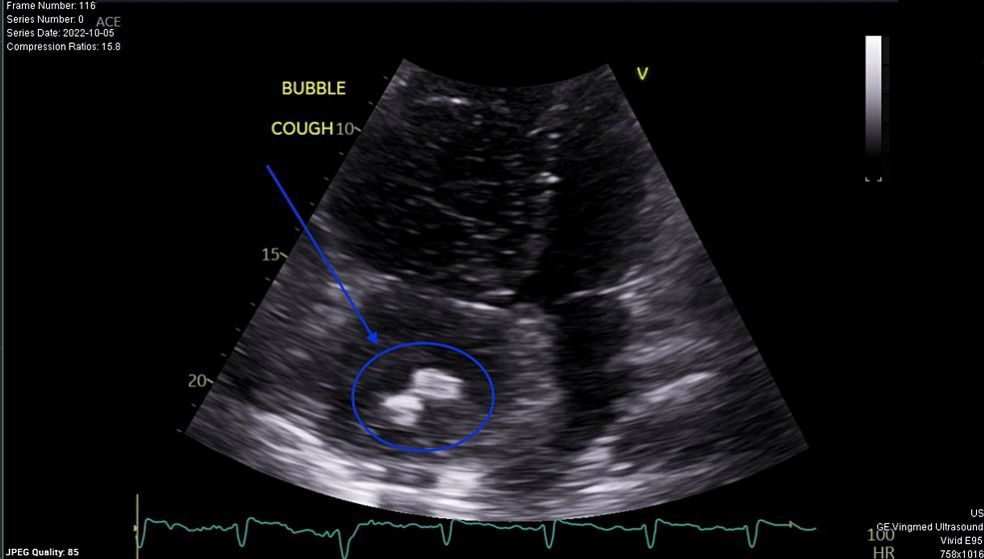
Persistence of right atrial mass post-thrombolysis with alteplase on repeat transthoracic echography.
